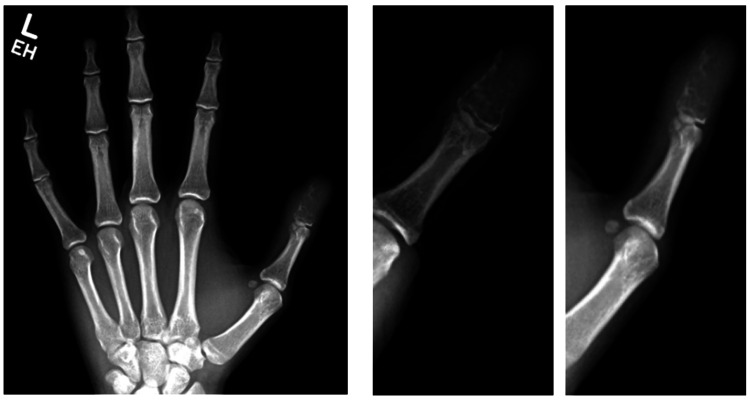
Preoperative X-rays of the left hand and thumb Images show an expansile, lytic lesion of the left thumb distal phalanx with cortical erosion.
